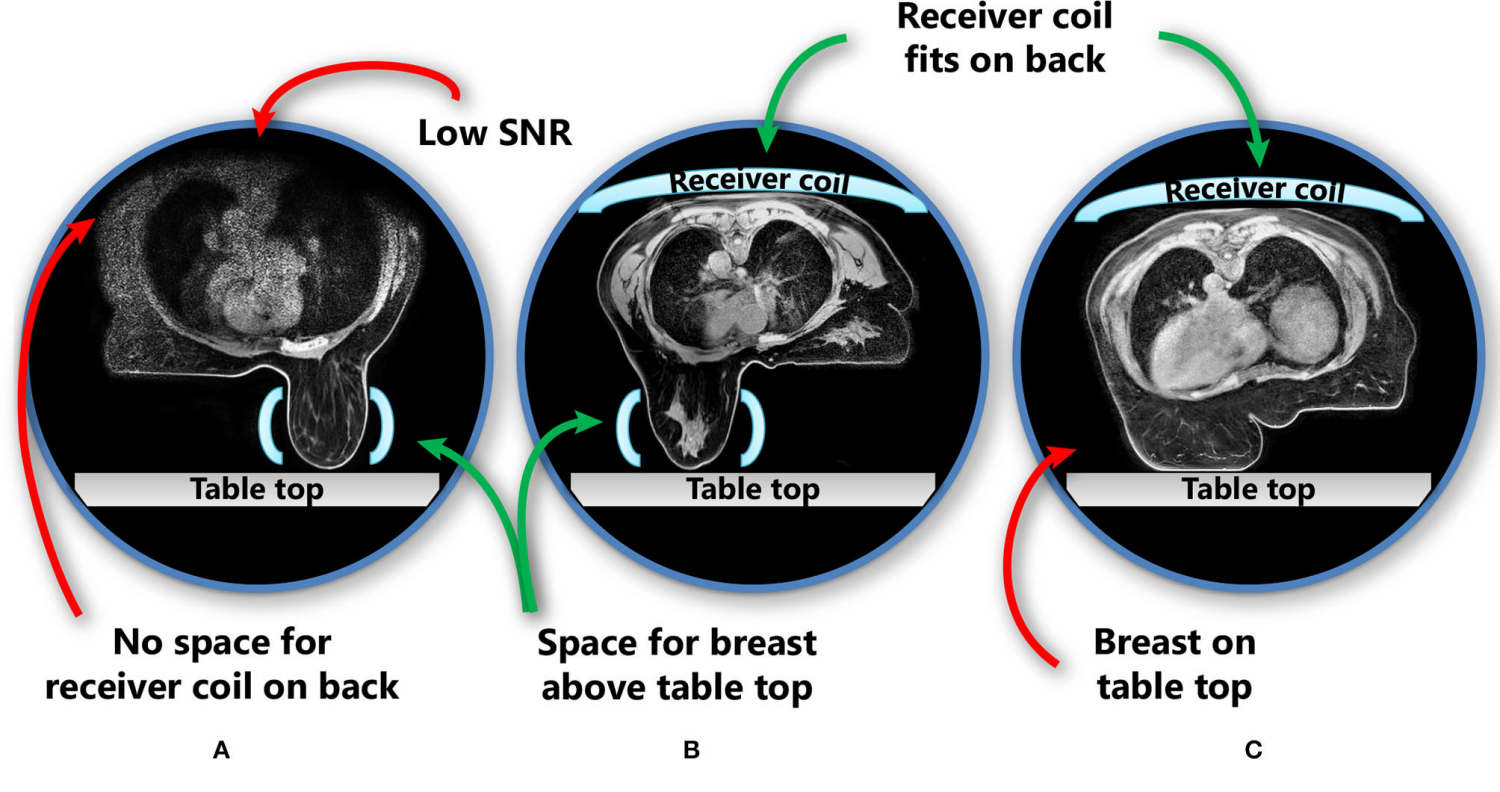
Patient and receiver coil positioning in prone position, including challenges in this position. The images show three different patients. (A) No space for the receiver coil on the back of the patient if the breast hangs freely without touching the scanner table; (B) the receiver coil fits above the patient while also the breast hangs freely; (C) when the receiver coil is fitted in the MRI bore above the patient, the breast touches the table top and is deformed. Light blue shapes represent the receiver coils (horizontal: receiver coil array; vertical: single flex coil). SNR, signal-to-noise ratio.
